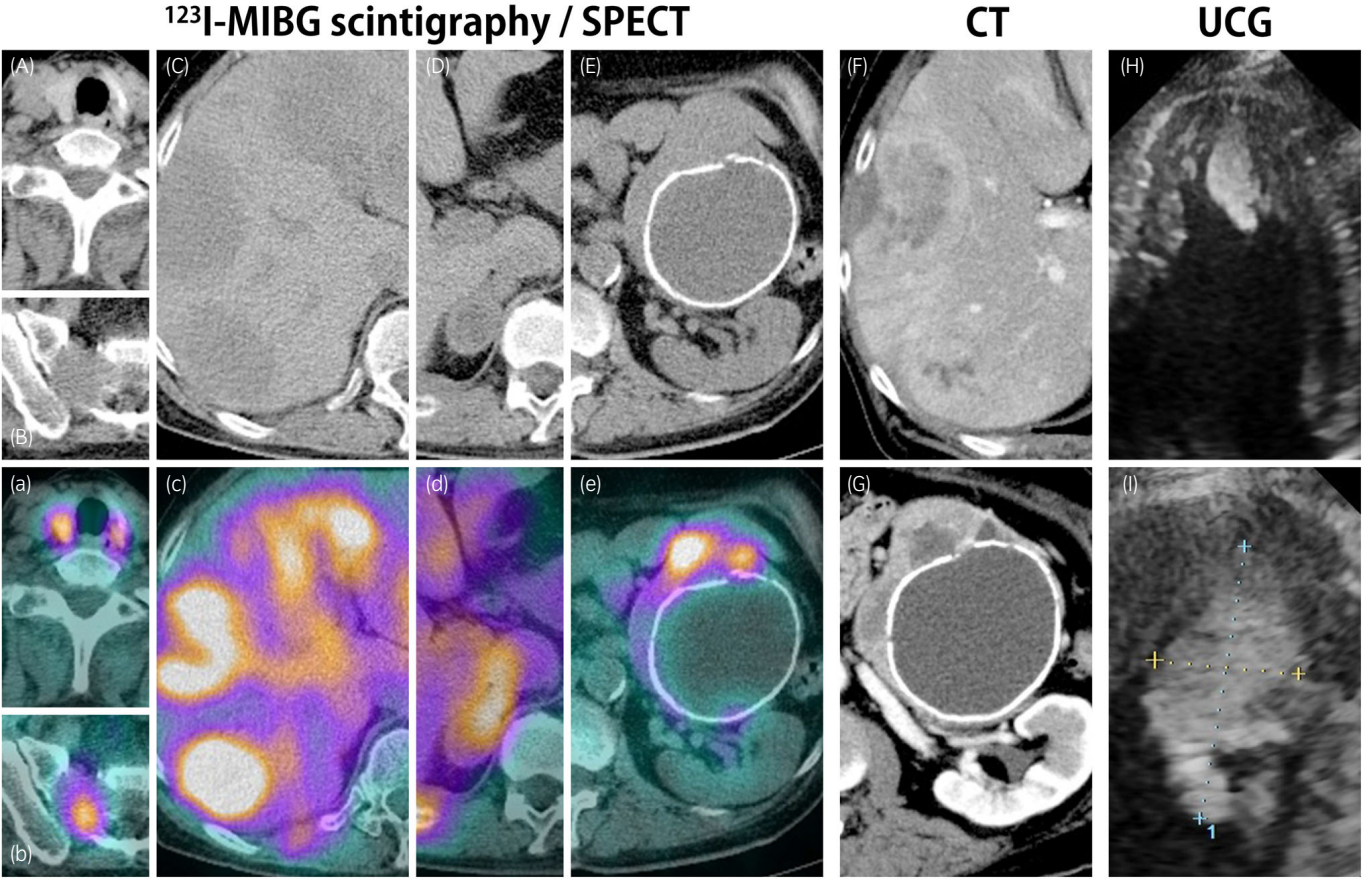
Imaging study of the present case, SIMC‐Uro #11,956*. CT without contrast (A‐E) and ^123^I‐metaidobenzylguanidine (MIBG) scintigraphy (a‐e) and CT with contrast (F and G) on admission, showing the multiple accumulations of MIBG in the thyroid, liver, bones, bilateral adrenal glands, and sacrum, and the left adrenal gland with a size of 120 mm accompanied by internal calcification and a cystic lesion. Cardiac ultrasonography (H and I) showing a 28‐mm hyperechoic embolus at the apex of the heart. *: A unique non‐sequential patient control number in the Department of Uro‐oncology, Saitama Medical University International Medical Center.
